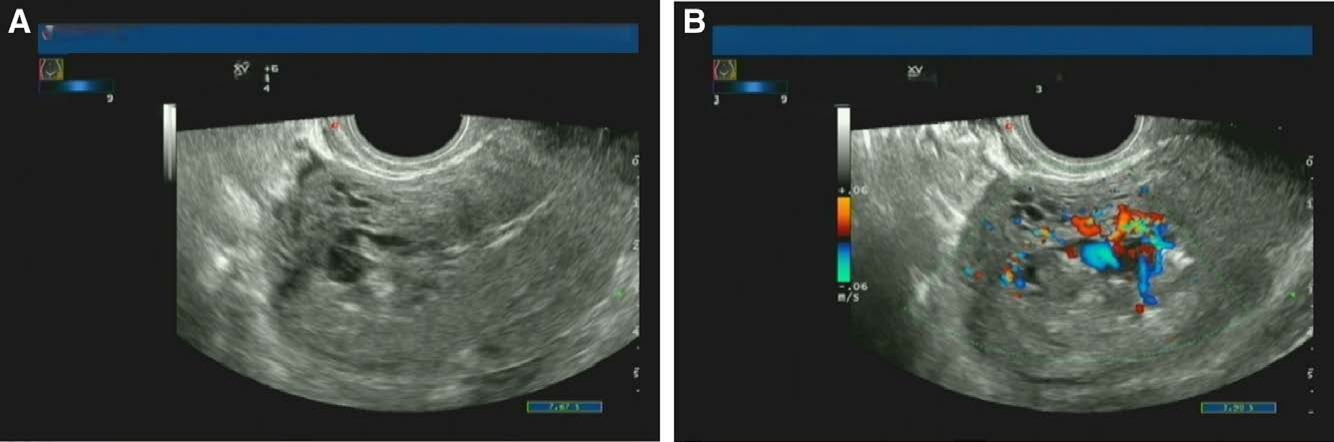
The ultrasound image shows uterine arteriovenous fistula (UAVF). The ultrasonic image showed an inhomogeneous echo mass at the right side of the uterine horn, about 3.5 × 3.1 cm in size, with unclear boundary and uneven internal echo. Many tortuous and dilated vascular echoes could be seen inside (A), and arterial and venous blood flow signals could be detected (B).
